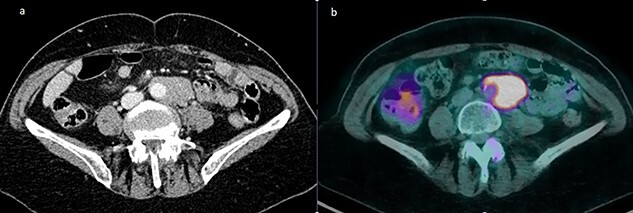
(a) Solid tissue enveloping the terminal portion of the abdominal aorta measuring approximately 45 × 26 × 81 mm in diameters; (b) fluorodeoxyglucose PET showing a pathological uptake with SUV of 10 at the level of the para-aortic lymphomatous tissue.
